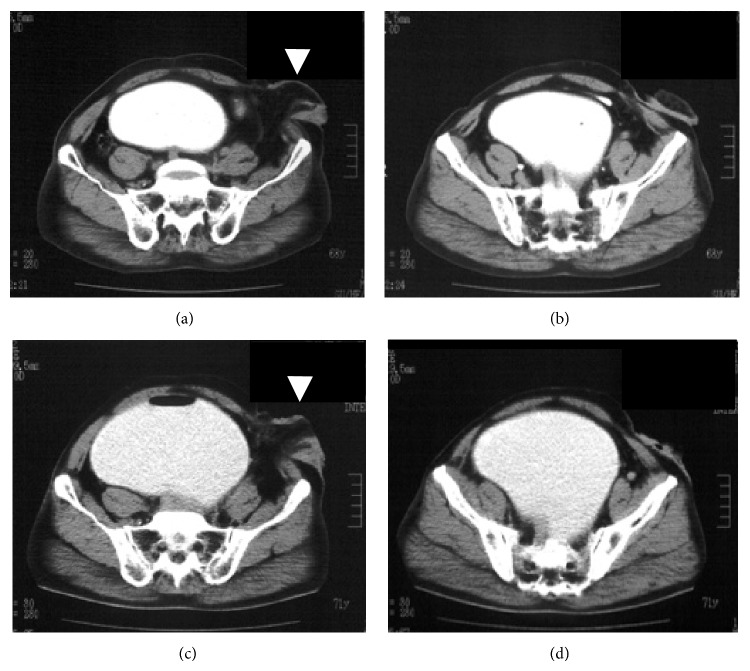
Computed tomography (CT) revealed a large cystic mass in the pelvic cavity at our surgical outpatient clinic ((c), (d)) and at another hospital two years previously ((a), (b)). A sigmoid divided colostomy was created in the left lower abdomen (arrow head).
